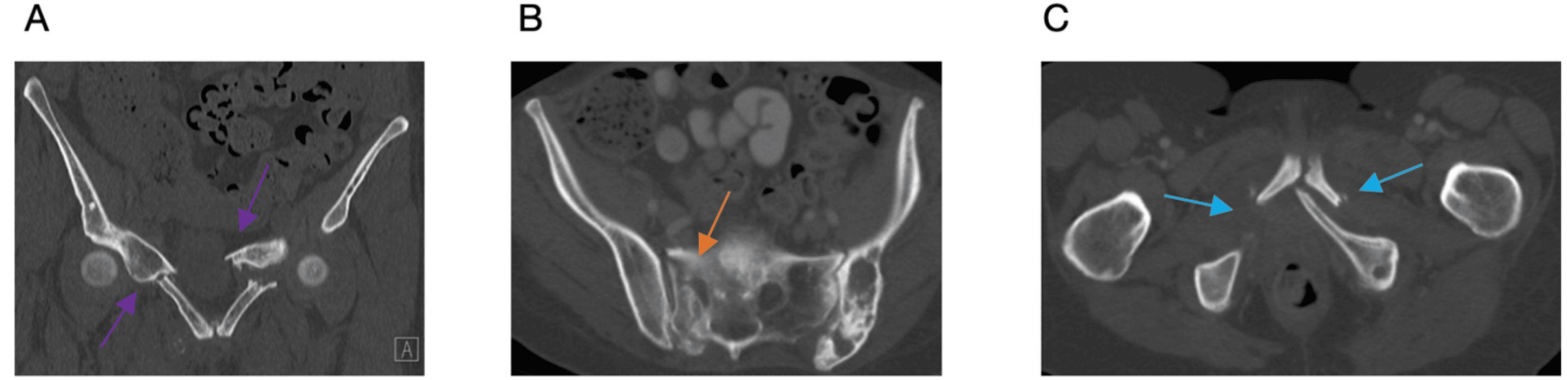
Computed tomography (CT) for surgical planning Image A demonstrates bilateral pubic ramus fractures (purple arrows). Image B demonstrates zone I sacrum fracture (orange arrow). Image C demonstrates bilateral parasymphyseal fractures (blue arrows).
